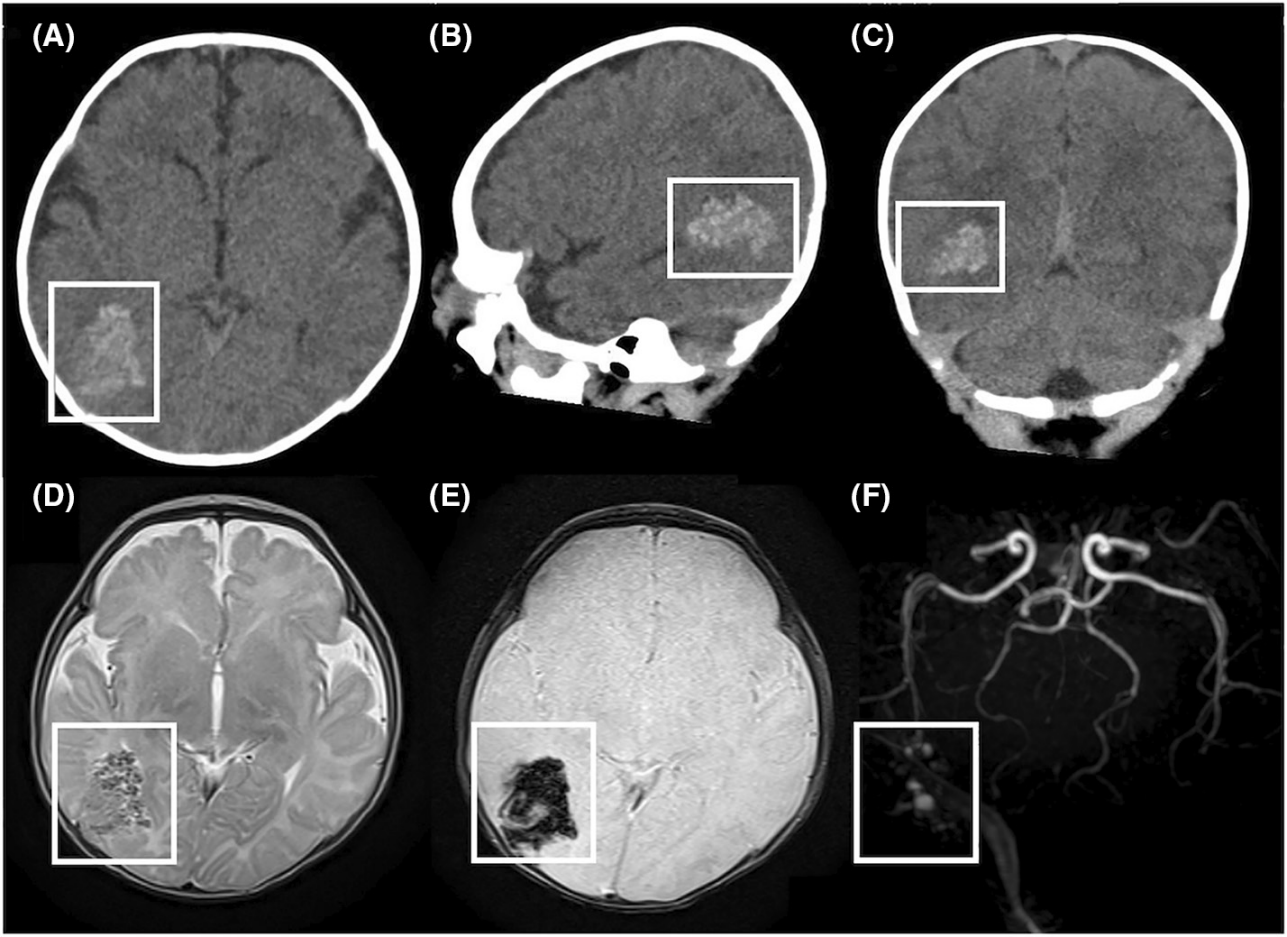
(A–C) Computed tomography. (A) Axial view. (B) Sagittal view. (C) Coronal view. The lesion was hyperdense and located in the right occipitotemporal region (white box). (D–F) Magnetic resonance imaging. (D) T2‐weighted sequence. (E) T2‐star‐weighted sequence. (F) Magnetic resonance angiography. The lesion appeared as a cluster of low signal‐intensity nodules on T2‐weighted images and a low signal‐intensity lesion on T2‐star‐weighted images. No obvious feeder vessel was identified on magnetic resonance angiography.
